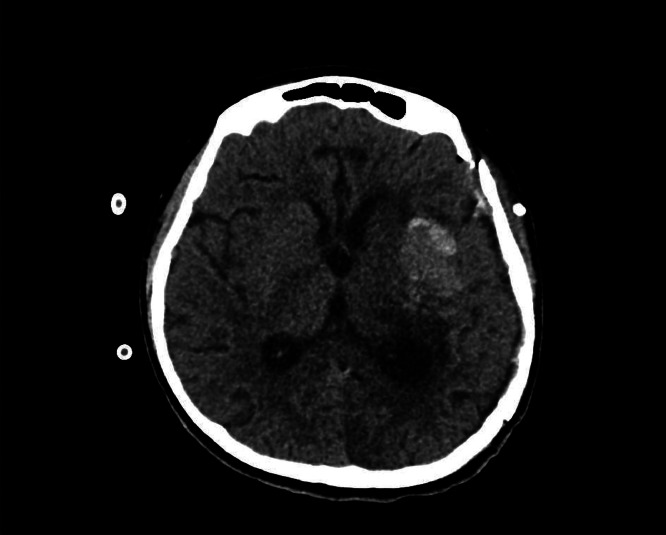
Acute left basal ganglia hemorrhage with perifocal edema and mass effect. This non-contrast axial CT scan of the brain demonstrates an acute intracerebral hemorrhage (ICH). A well-defined hyperdense lesion is present in the left basal ganglia region, consistent with acute blood. Surrounding the hematoma, there is hypodensity suggestive of perifocal edema. Mild mass effect is evident, as indicated by the effacement of the adjacent sulci and compression of the right lateral ventricle. No significant midline shift is observed at this level.
